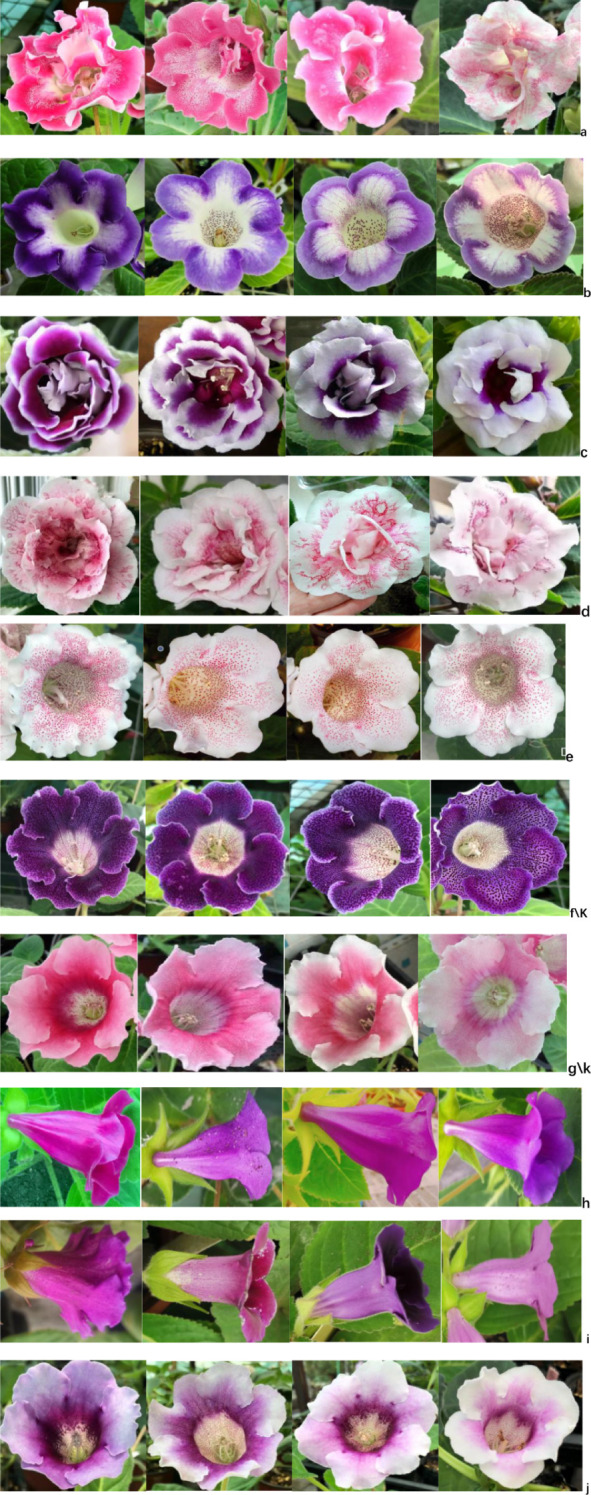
Corolla characteristics on inbred progeny genetic expression. (a) HDP (b) RR (c) WE (d) PM (e) MO (f/k) S + ST (g/k) SM + ST (h) LLCT (i) SLCT (j) CT SLCT (j) CT.
